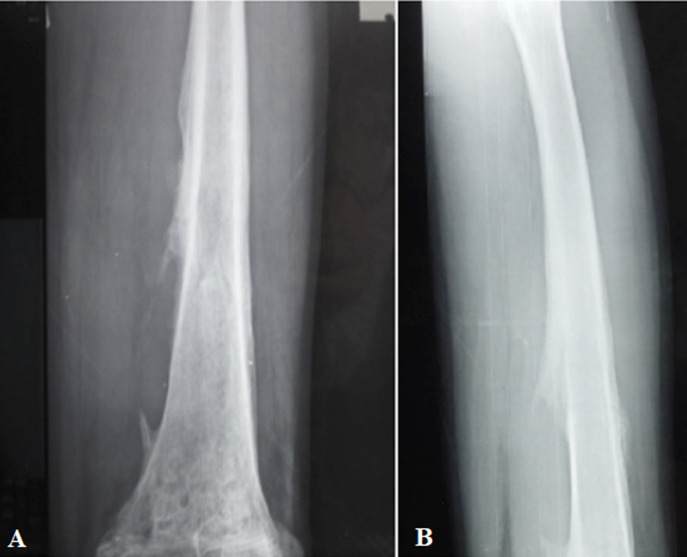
(A, B) anteroposterior and lateral X-ray of the left femur showing a moth-eaten osteolysis in the distal metaphysis extending to the epiphysis and the diaphysis, with periosteal reaction type Codman triangle and edema of the surrounding soft tissues
